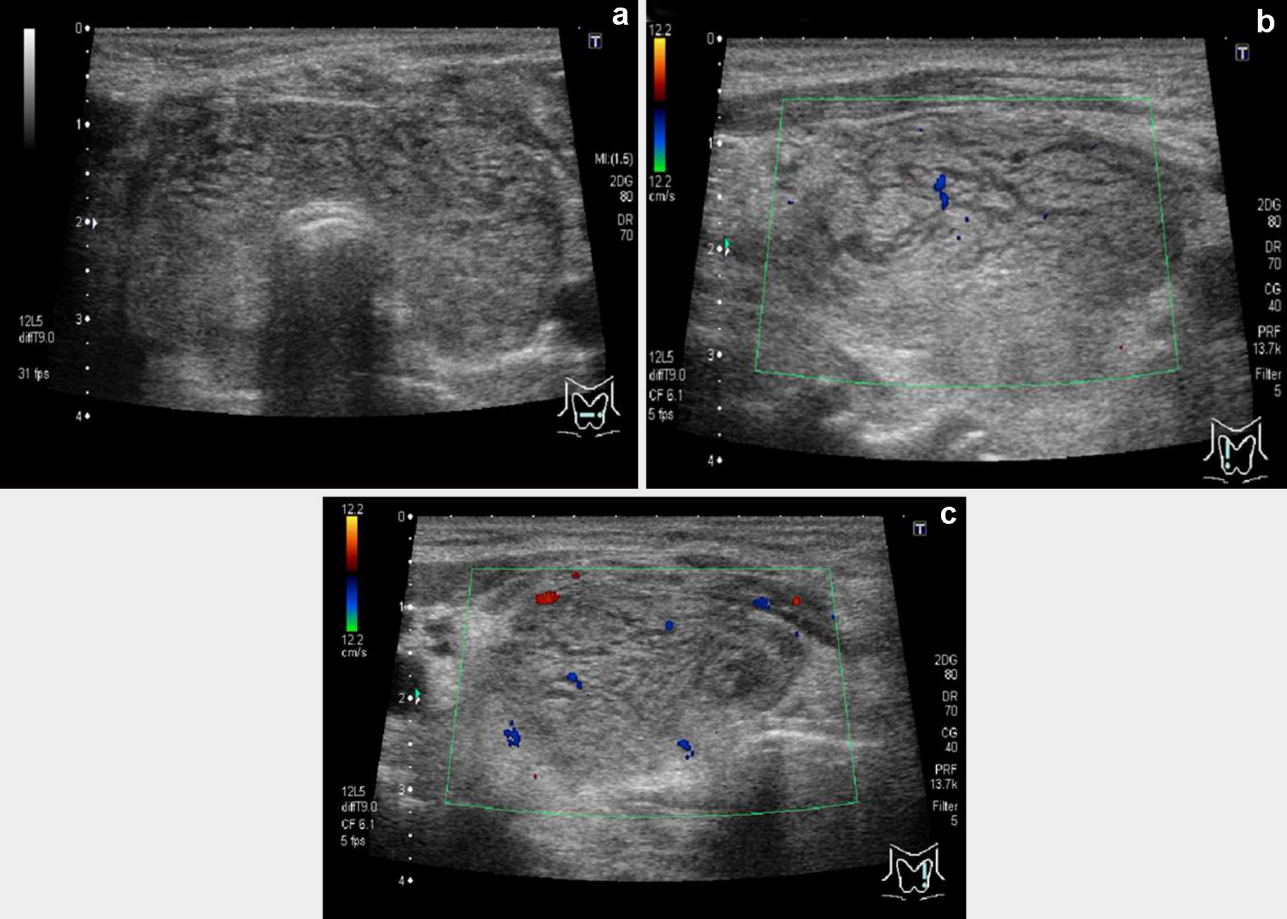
Thyroid ultrasonography after admission. a Transverse section of the thyroid gland on B-mode (brightness mode) ultrasound imaging. The thyroid was diffusely enlarged, with a 10-mm-thick isthmus. No nodular lesion was observed. The thyroid parenchyma was not of uniform echogenicity, with many hypoechoic reticular lines scattered in it. b Longitudinal section of the right lobe of the thyroid on color Doppler ultrasound imaging. c Longitudinal section of the left lobe of the thyroid on color Doppler ultrasound imaging. A Doppler signal was not detected in the hypoechoic reticular lines
